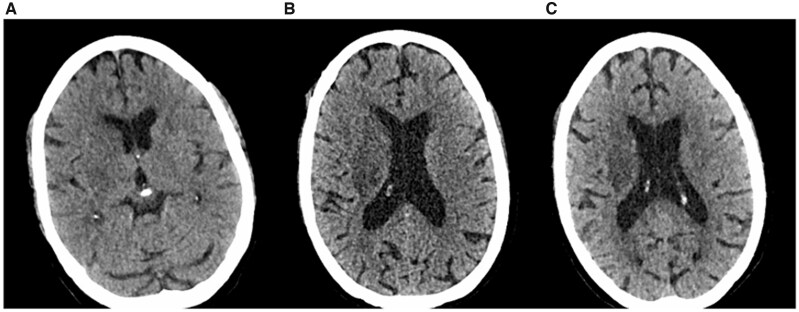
Progression of infarct. (A) CT head taken 1 day post-admission showing evolving ischaemia in lenticulostriate territory. (B) CT head taken 6 days post-admission showing development of this ischaemia. (C) CT head taken at 13 days post-admission showing further, expected evolution.
